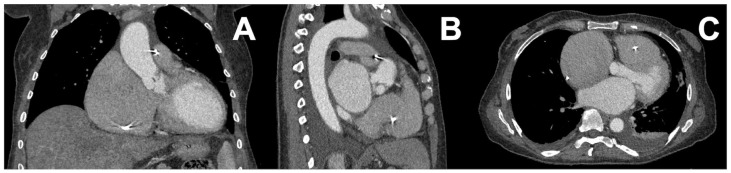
(A) Coronal, (B) Sagittal reformatted images from a dedicated cardiac CT acquisition. (C) Representative axial slice through the heart. These data are the foundation of planning an endoscopic minimally invasive valve repair. This patient underwent triple (aortic, mitral, tricuspid) valve repair/replacement surgery. Using a thin slice image set (<1.25 mm slice thickness) the regions of interest can be segmented for 3D printing and other virtual planning using CAD.
